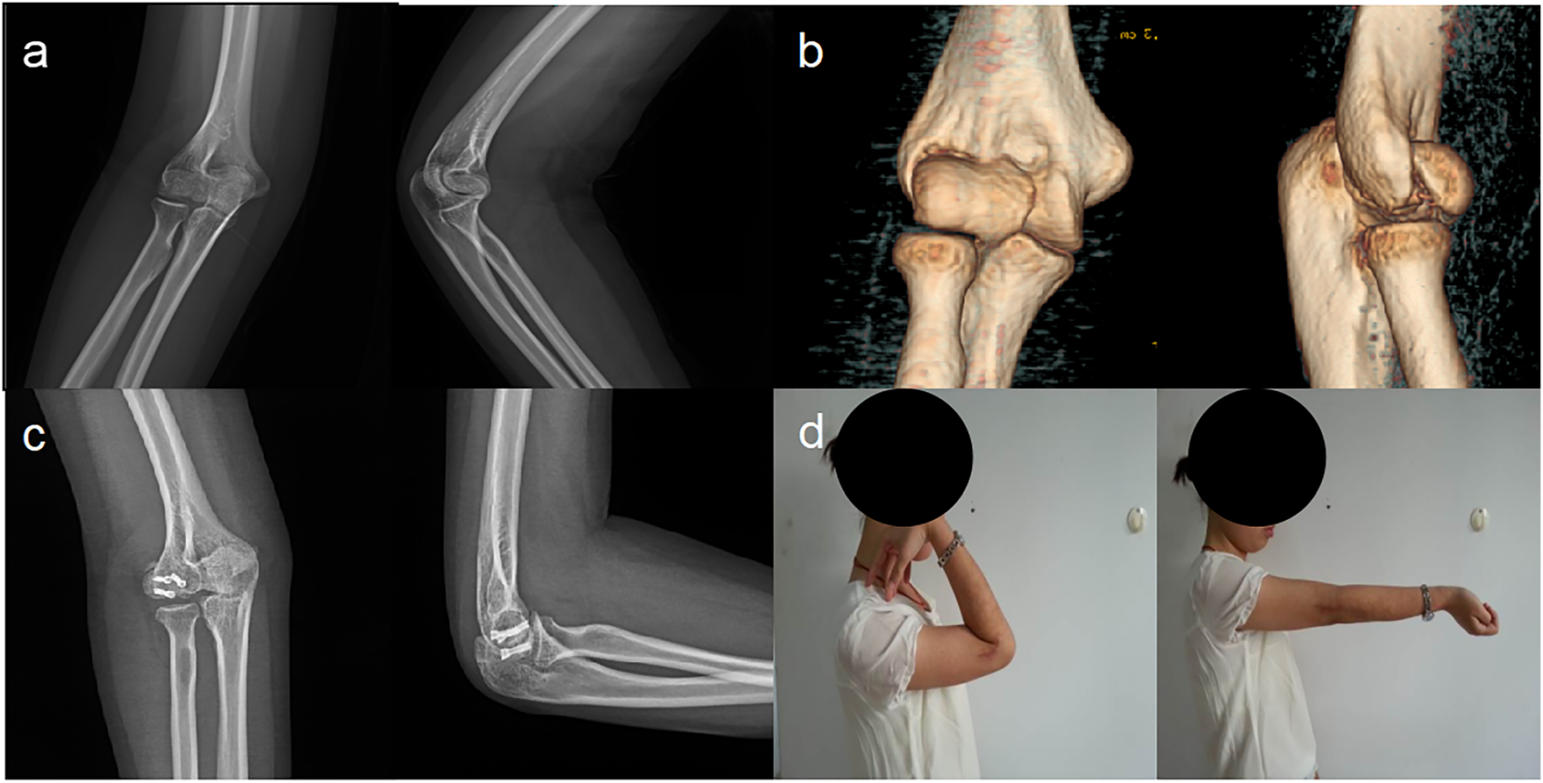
Dubberley type 2 fracture: a A 30-year-old female had a coronal shear fracture of distal humerus (Dubberley 2A) with preoperative X-ray images of distal humerus. b Preoperative CT images showed intact fragment of capitellum and trochlea. c Postoperative X-ray images showed three headless compression screws for fracture fixation. d Functional outcome was evaluated to be good at last visit at least 1 year following-up.
